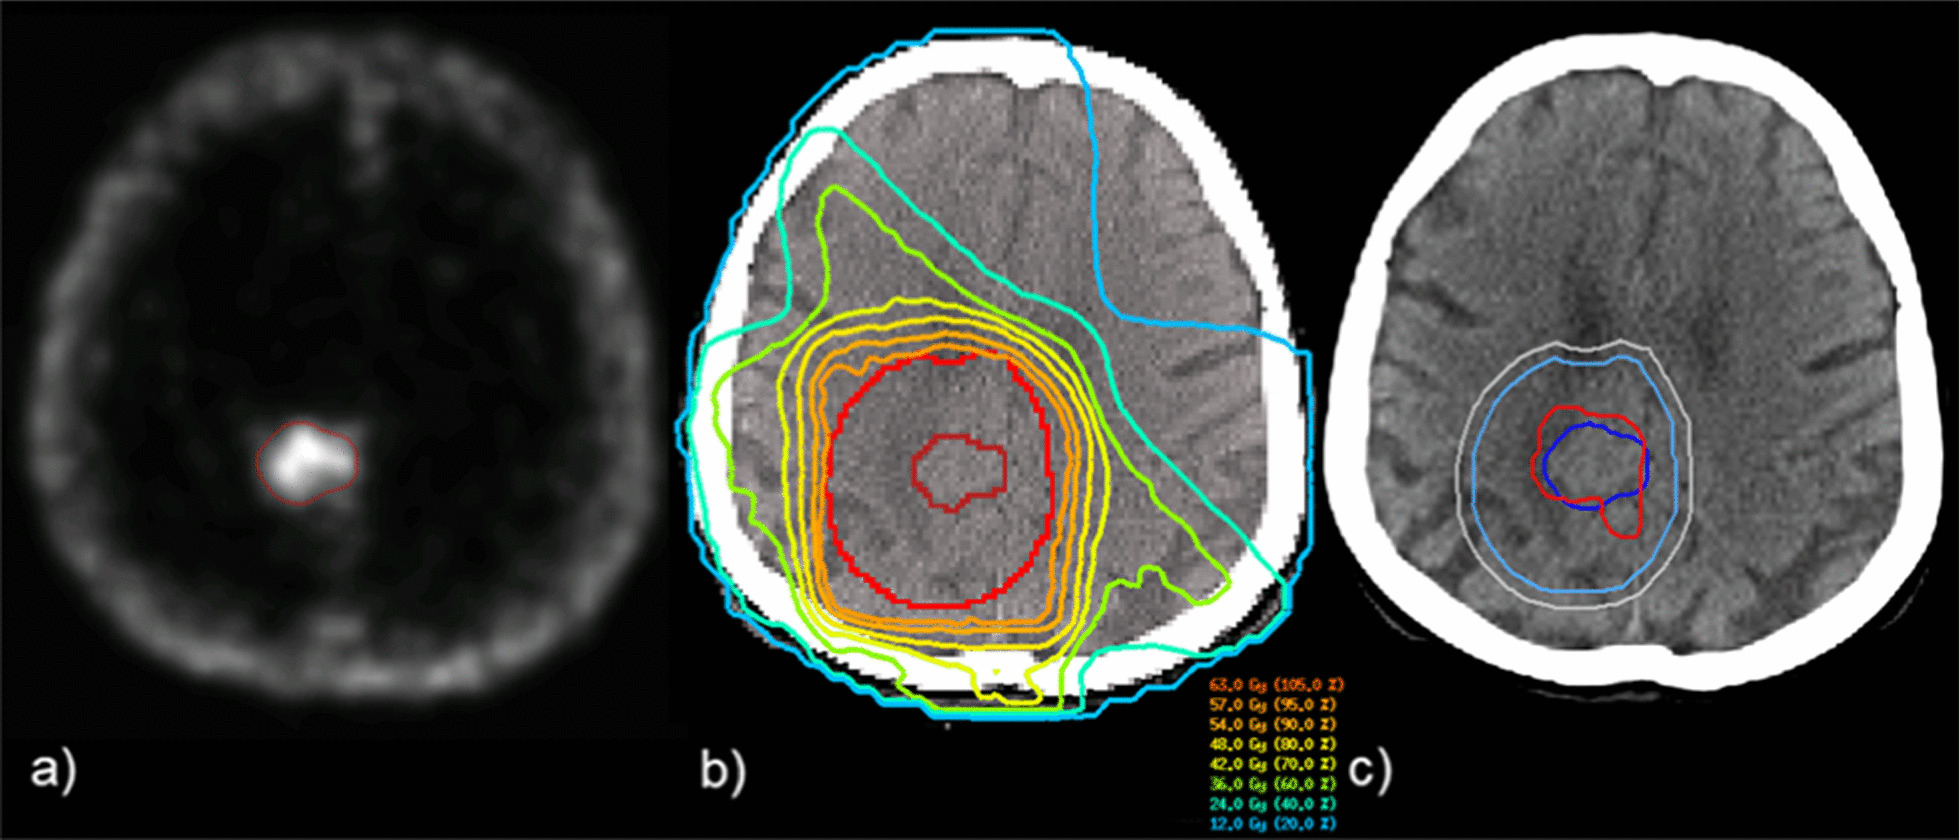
Clinical example for a SSTR-PET-CT imaging with high tracer uptake exhibited by the meningioma (red); b dose distribution as delivered during treatment (GTV (dark red), PTV (red)); c delineation of GTVini (blue) and GTVrt (red), CTV (light blue) and PTV (grey)
